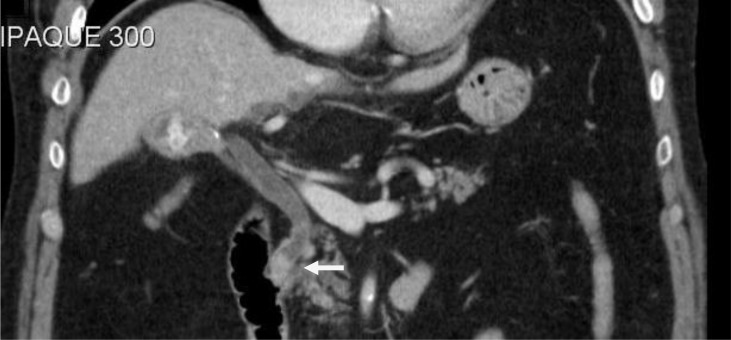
Coronal computed tomography demonstrating a stone in the gallbladder and a stone at the lower end of a distended common bile duct
